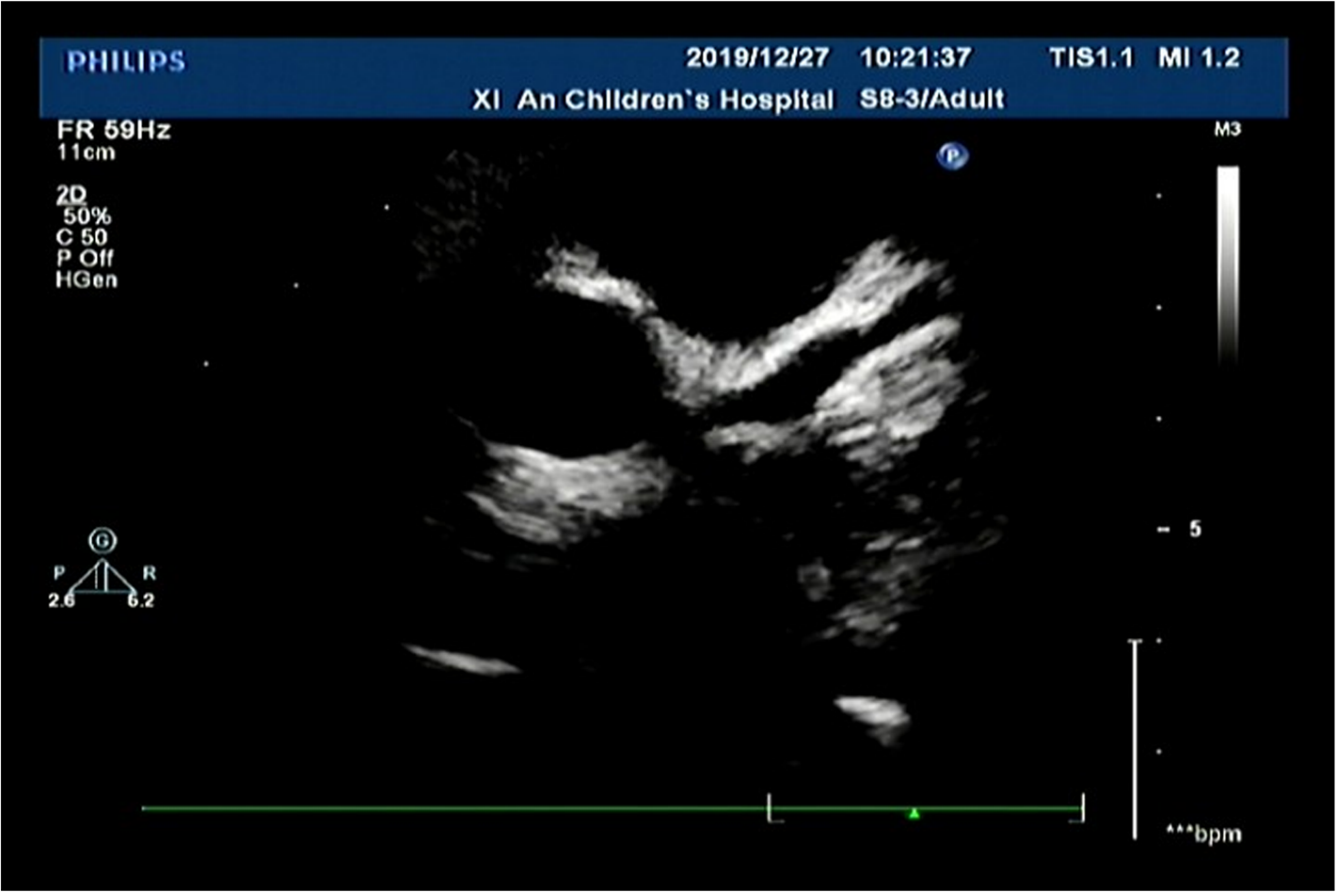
The doppler echocardiographic after 60 days onwards
